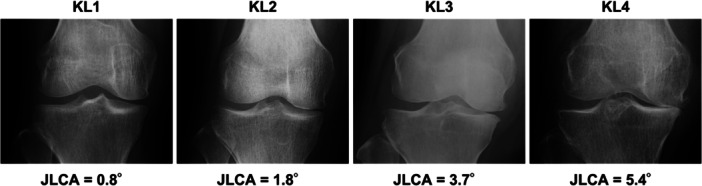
Representative radiographs of Kellgren–Lawrence grade and joint line convergence angle. JLCA, joint line convergence angle; KL, Kellgren–Lawrence grade.
